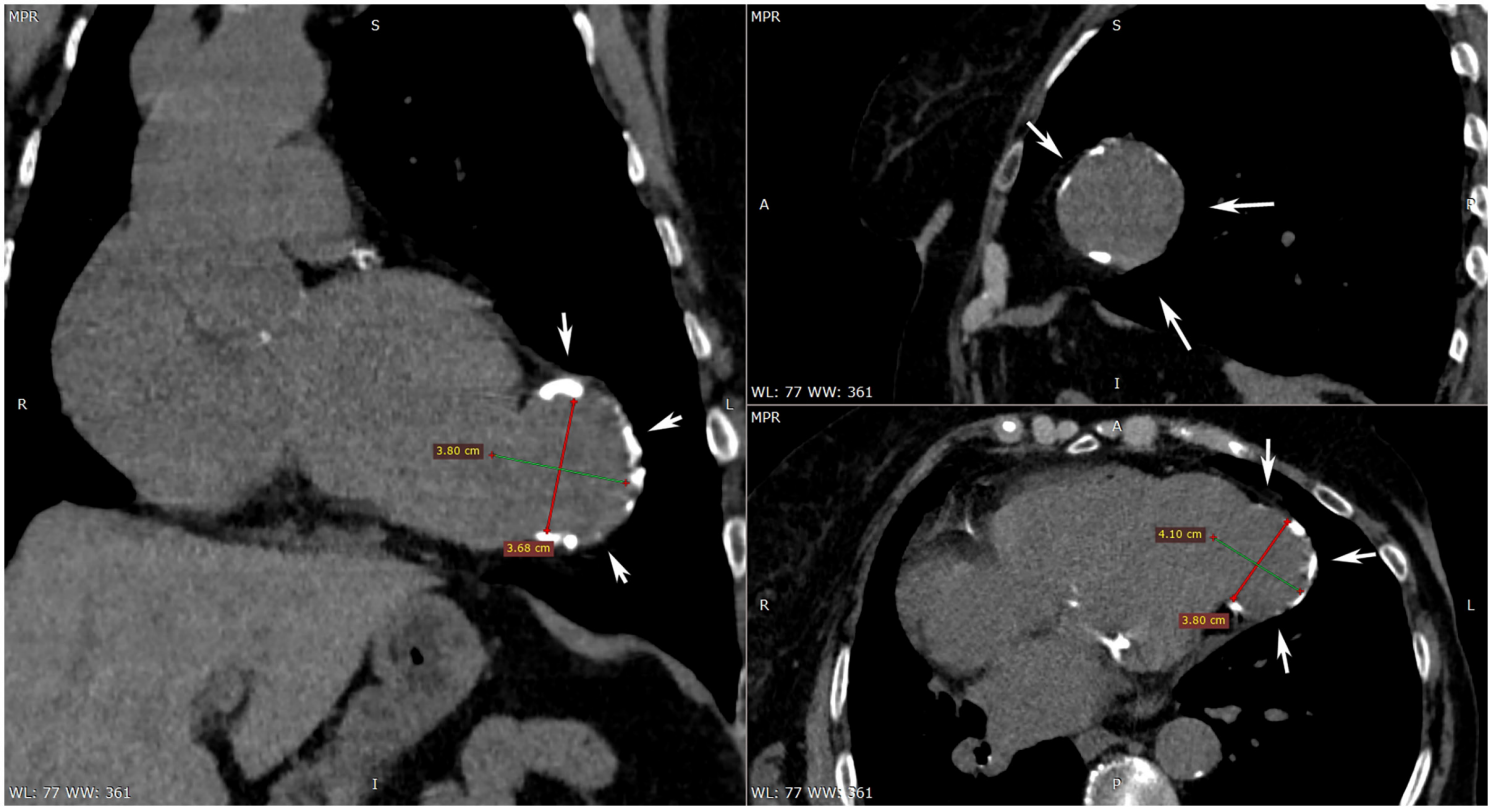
CT images of the aneurysm in oblique, sagittal, and axial view, with diameter measurements
